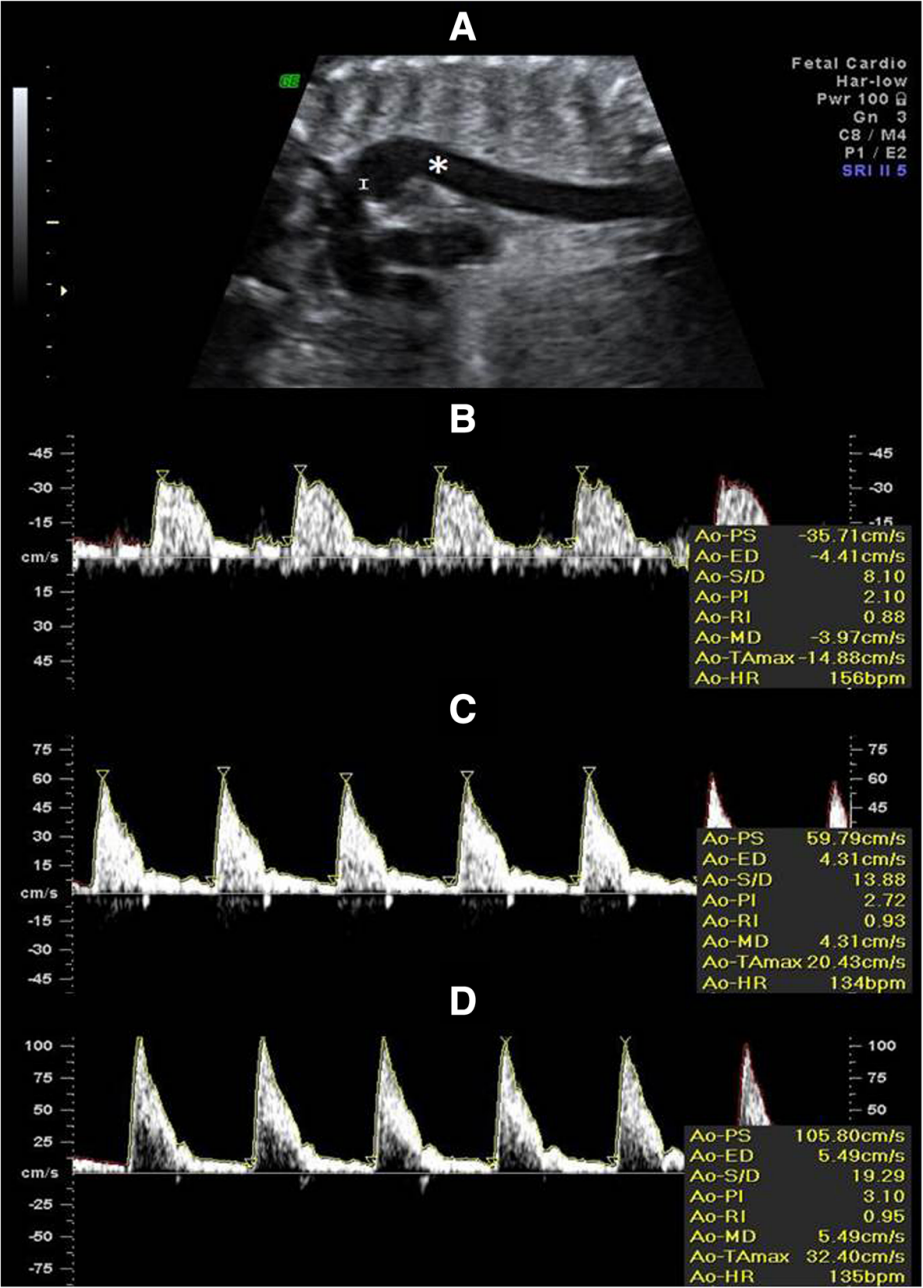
Ultrasound representative images of the foetal aorta. (A): Aortic arch showing the position of the aortic isthmus (I) and the proximal descending aorta (*). (B), (C) and (D): Doppler flow waveforms at the first (B), second (C) and third (D) trimesters, respectively. The flow profile in the foetal aorta is characterized by a steep systolic rise, a postsystolic notch and a low end-diastolic forward flow velocities. The acceleration time is a reflection of cardiac contractility, while the subsequent diastolic phase reflects peripheral vascular impedance. The postsystolic notch develops as a result of aortic valve closure during early ventricular diastole resulting in a slight decrease in flow velocity.
